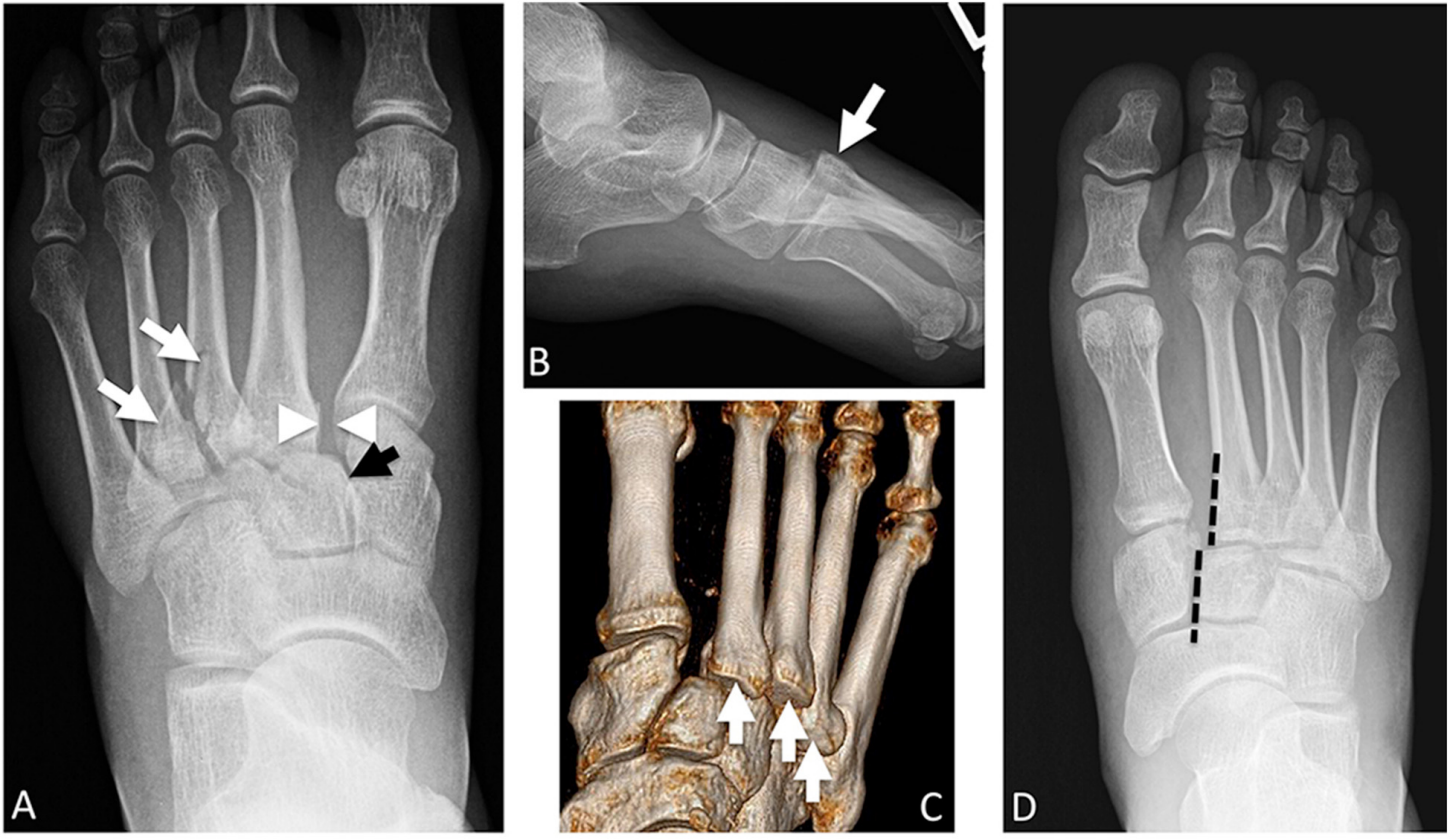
Lisfranc fracture-dislocation. a AP view of the foot with widening of the space between the first and second metatarsal bases (*arrowheads*). Misalignment of the second metatarsal base from the middle cuneiform (medial margin of middle cuneiform demarcated with *black arrow*). Fractures of the bases of the third and fourth metacarpals (*white arrows*). b Lateral view in the same patient shows dorsal displacement of the second to fourth metatarsal bases (*arrow*). c Volume rendered CT reformat in a different patient with dislocation of the second, third, and fourth metatarsal bases. d Different patient. Additional Lisfranc fracture dislocation. Here, the misalignment of the second metatarsal base from the middle cuneiform is shown with *dashed lines*—these should be in the same line. Again, there is widening of the first and second metatarsal base interspace
